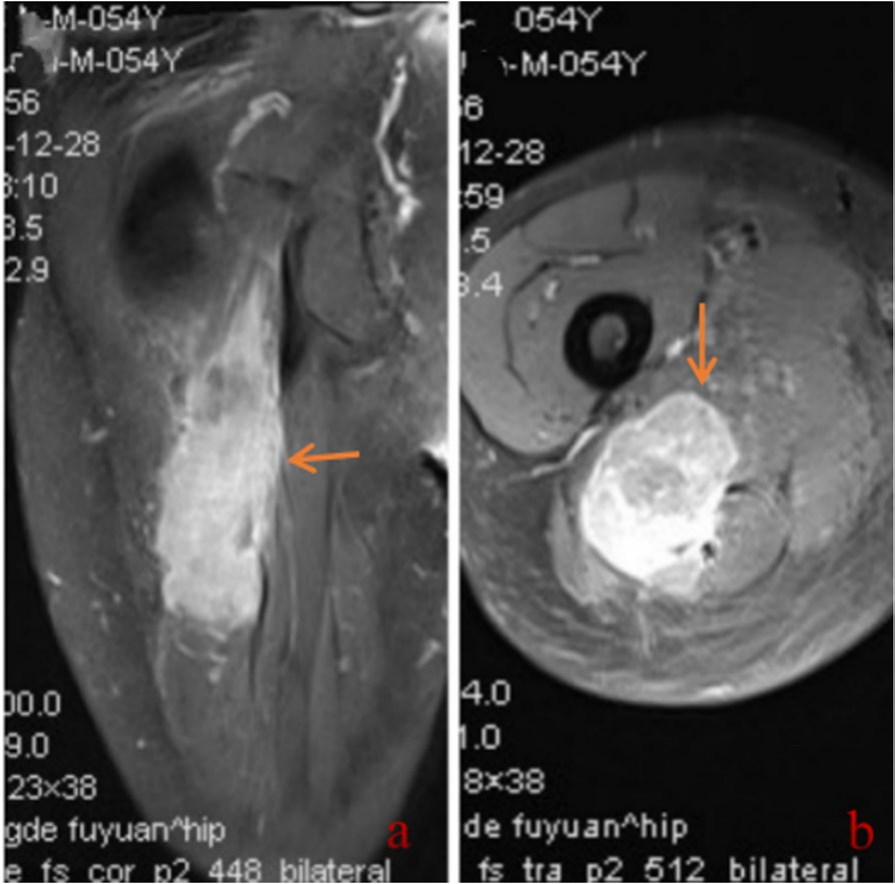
Gluteal MRI in case 1. a Coronal MRI shows that the right thigh tumour appeared as an intramuscular mass with inhomogeneous vascularization (arrow). b T2-weighted axial MRI shows a mass with high signal intensity (arrow)
